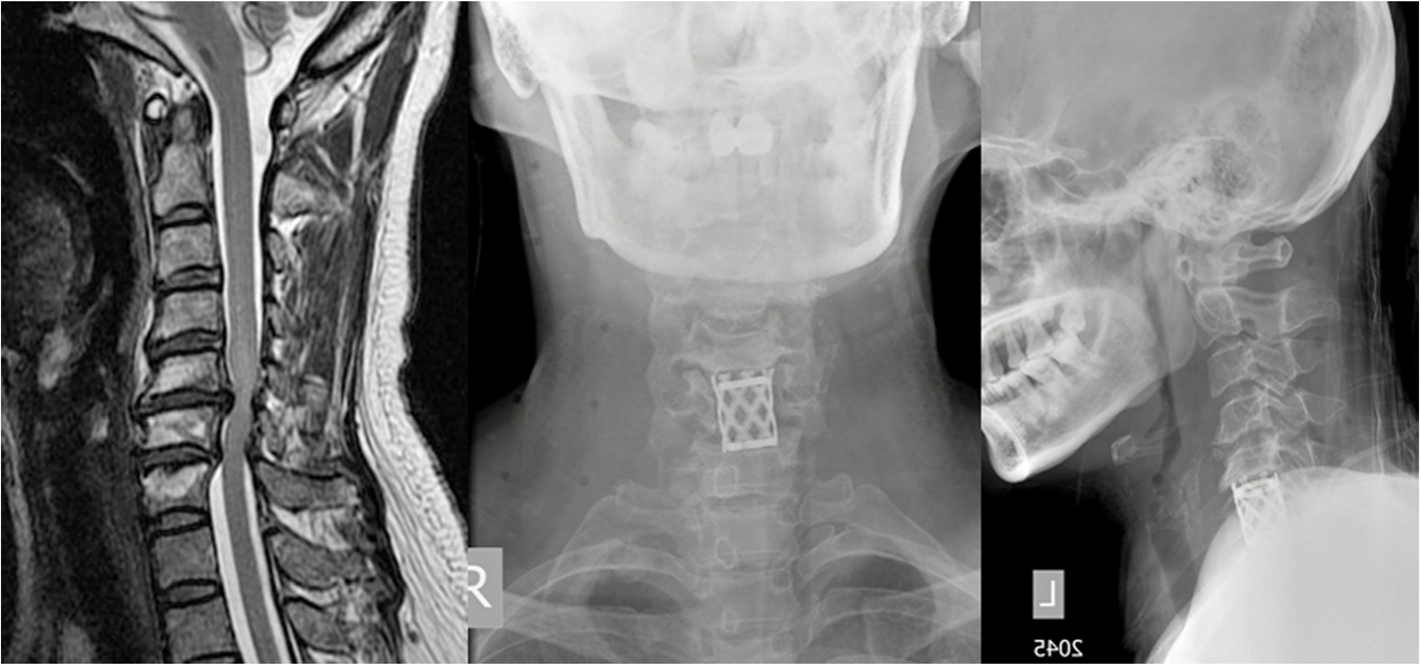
T2 weighted MRI image showing a patient with cervical spondylotic myelopathy involving C5-C6 and C6-C7 levels (left) who underwent an anterior cervical corpectomy and fusion from C5-C7. Post-operative AP (middle) and lateral (right) x-ray images show a cage with bone graft stabilizing the C5-C7 segments
